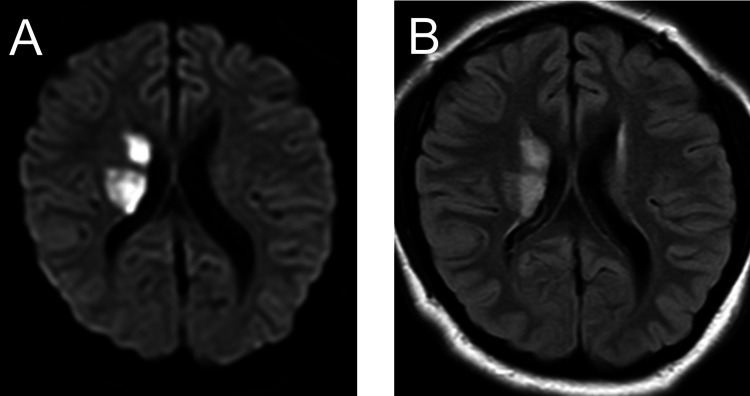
Brain magnetic resonance imaging (A) Diffusion-weighted imaging and (B) fluid attenuation inversion recovery imaging show a high-intensity lesion involving the right putamen, anterior limb of the internal capsule, and part of the head of the caudate nucleus.
